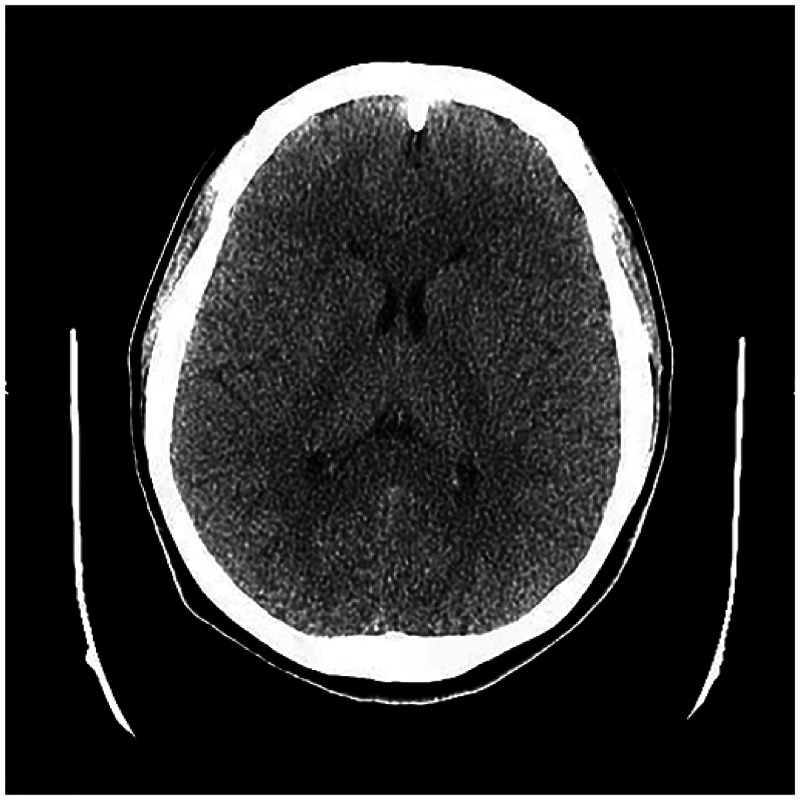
Computed tomography scans of the head (March 2021) reveal no abnormalities.
